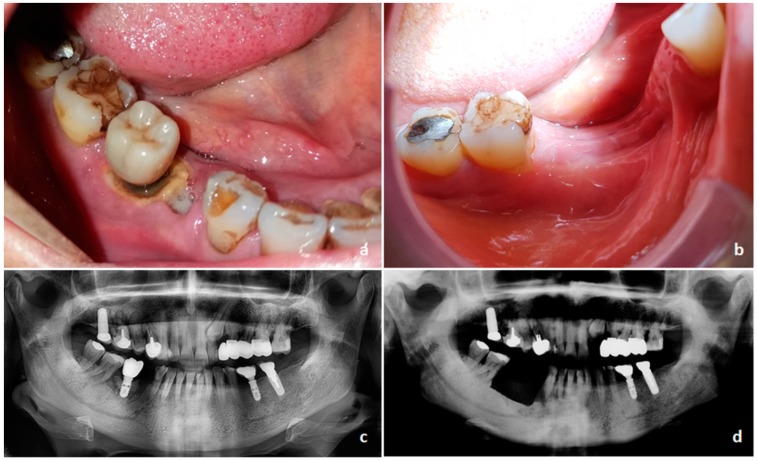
Clinical aspect of classical exposed bone variant of osteonecrosis of the jaw (ONJ). (a) Intraoral view of exposed and necrotic bone around a dental endosseous implant, together with infected post-extraction socket of dental element 45, with purulent discharge. Due to multiple myeloma, the patient had received zoledronate one year before. Extraction of tooth 45 was the trigger factor for ONJ. (b) Preoperative panoramic radiograph showing absent bone remodelling of the 45-extraction socket. (c) Postoperative healed intraoral view after a marginal mandibulectomy for removing the diseased bone. Primary closure of the surgical site was obtained by mobilization of a muco-periosteal flap. (d) Postoperative panoramic radiograph showing changes to trabecular pattern-dense bone of right hemi-mandibular body.
